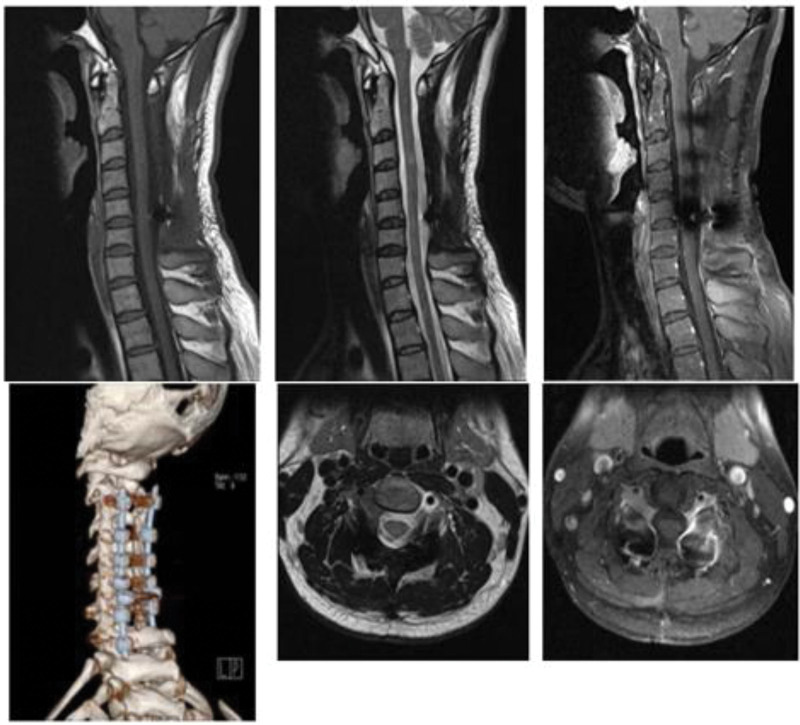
Postoperative magnetic resonance images 2 wk after surgery. The C2–C7 vertebral body and attachments are well retained with metal internal fixation. There is no loosening or fracture of the vertebral body and attachments, no occupation in the spinal canal, good morphology of the spinal cord without compression, and no abnormal enhancement on an enhancement scan.
